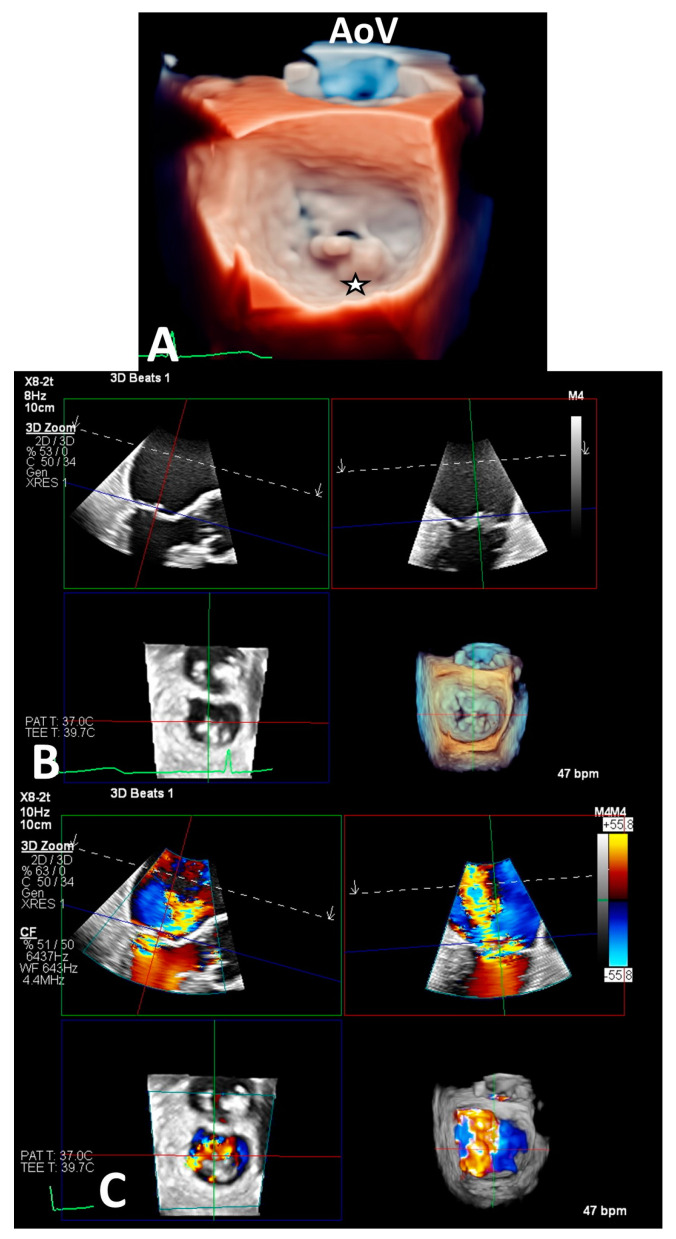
(A–C) depicts 3D en face imaging of the mitral valve. There is severe primary MR in the context of P2 prolapse and flail (A,B). The black star in (A) shows the site of pathology. AoV denotes the aortic valve for anatomic orientation. (C) shows color Doppler interrogation in 3D MPR revealing a significant anterolaterally directed regurgitant jet at the site of pathology.
